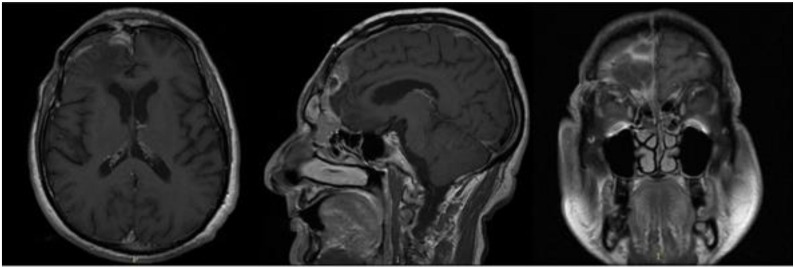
First recurrence of the lesion involving the cribriform plate (T1-weighted with contrast).
